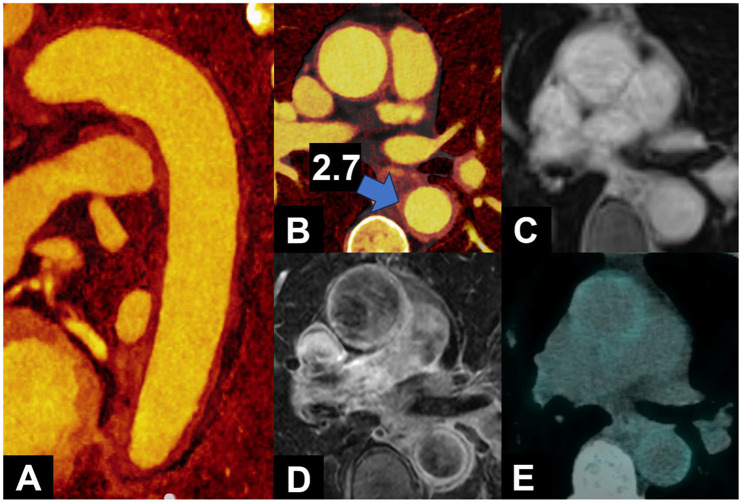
PCCT images of the thoracic aorta in a 69-year-old woman in venous phase. (A) and (B) color-coded iodine maps demonstrate circumferential wall thickening of the thoracic aorta with a quantitative iodine wall uptake of 2.7 mg I/mL. (C) T1-weighted late enhancement and (D) Dark blood images confirmed the circumferential wall thickening and increased gadolinium uptake of the thoracic aorta. (E) Subsequent ^18^ F-FDG-PET/CT confirmed increased tracer uptake indicative of large vessel vasculitis.
